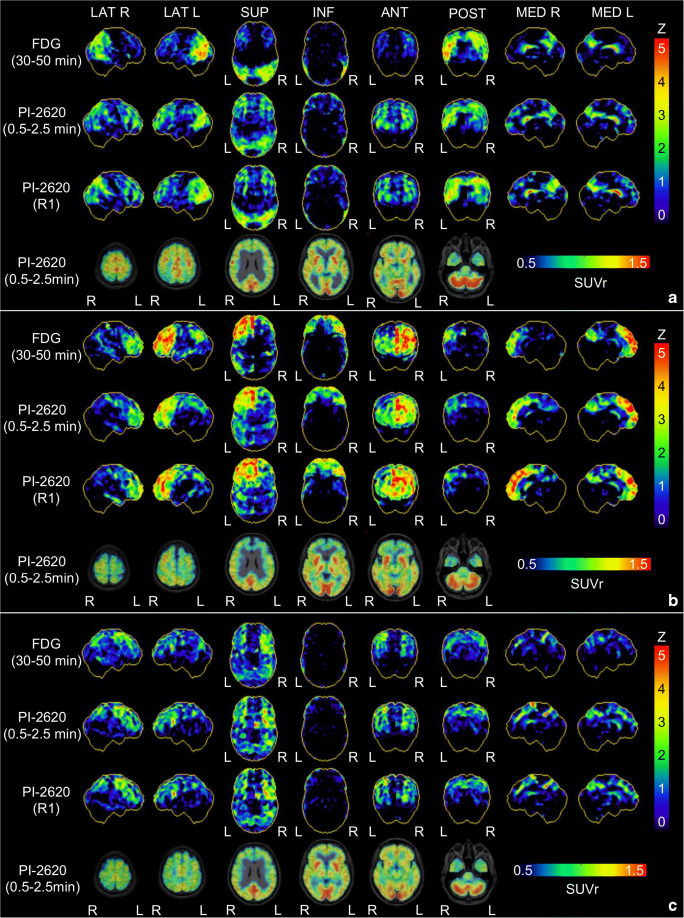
Representative 3D-SSP images (Z-score maps) of [^18^F]FDG-PET, early-phase [^18^F]PI-2620_0.5–2.5min_ and [^18^F]PI-2620_R1_ for three different most likely diagnoses of neurodegenerative disorders: a Alzheimer’s disease, b frontotemporal dementia, c progressive supranuclear palsy (Richardson syndrome). Surface projections from R, right; L, left; LAT, lateral; SUP, superior; INF, inferior; ANT, anterior; POST, posterior; MED, medial
